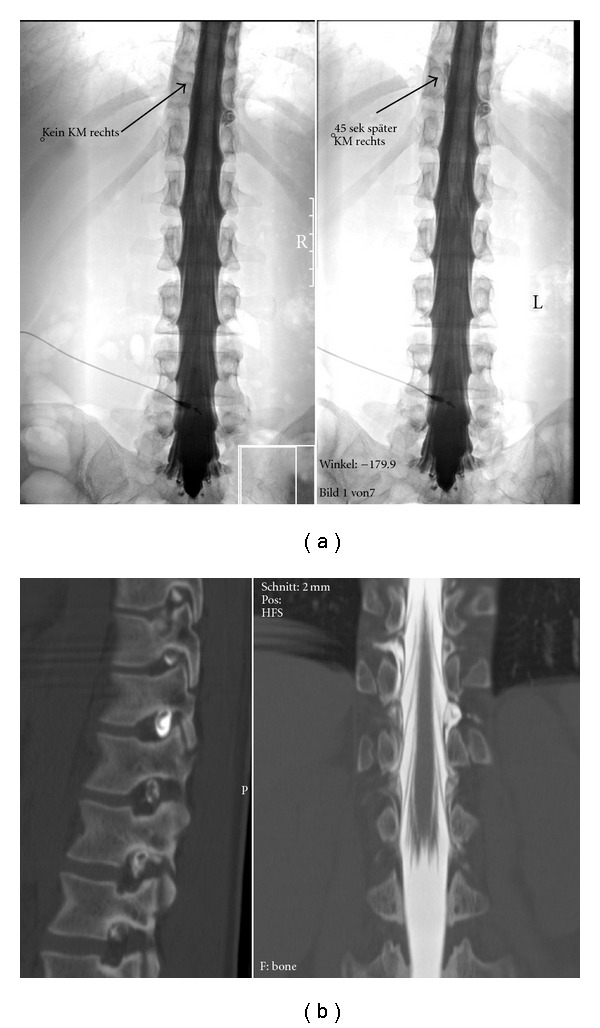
Spinal CSF leak causing subdural hematoma. (a) Left: contrast leakage to the left at the level of the D11 root. Right: 45 seconds later, contrast has flown around the dural sac and is exiting the spinal canal to the right. The dynamic series easily allows to study these flow dynamics and avoids misinterpretations. (b) Sagittal (left) and coronal (right) reformatted images from the subsequent myelo-CT show leakage in the left D11/12 foramen and contrast leakage to the right one segment above. This static study does not allow to exactly determine how contrast flows in and around the dural sac.
